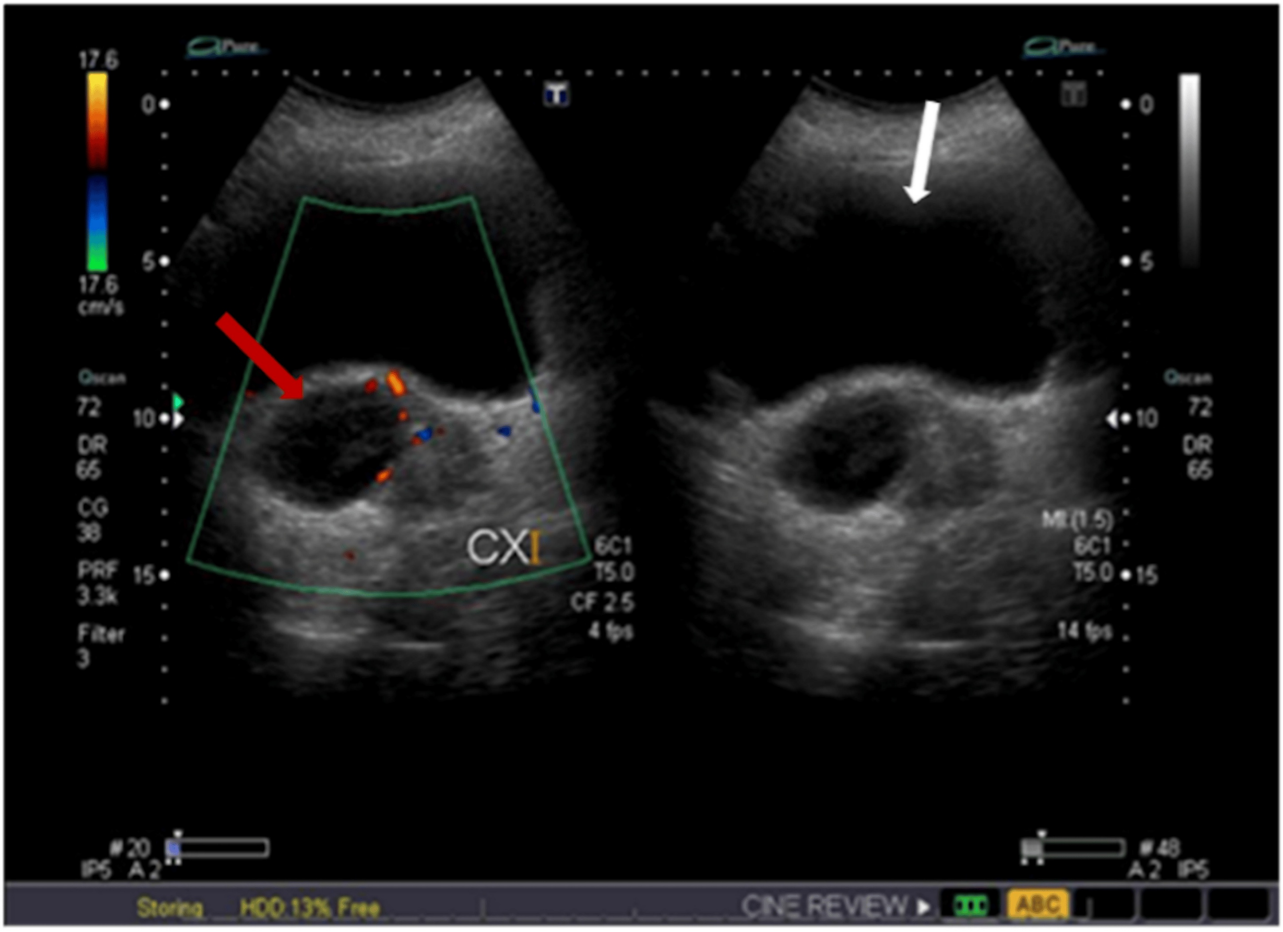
Targeted pelvic ultrasonography showing a vaginal cyst (red arrow) containing clear fluid, with no signs suggesting solid components or vascularity on Doppler imaging. The white arrow indicates the urinary bladder.
